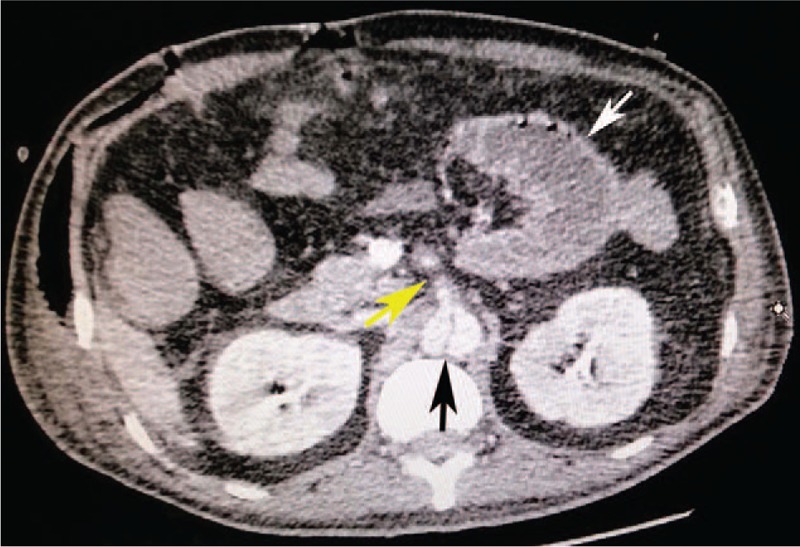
Computed tomography angiogram of the abdomen reveals aortic dissection involving the SMA (the black arrow), nonocclusive thrombosis of the SMA (the yellow arrow), and bowel wall edema (the white arrow).
